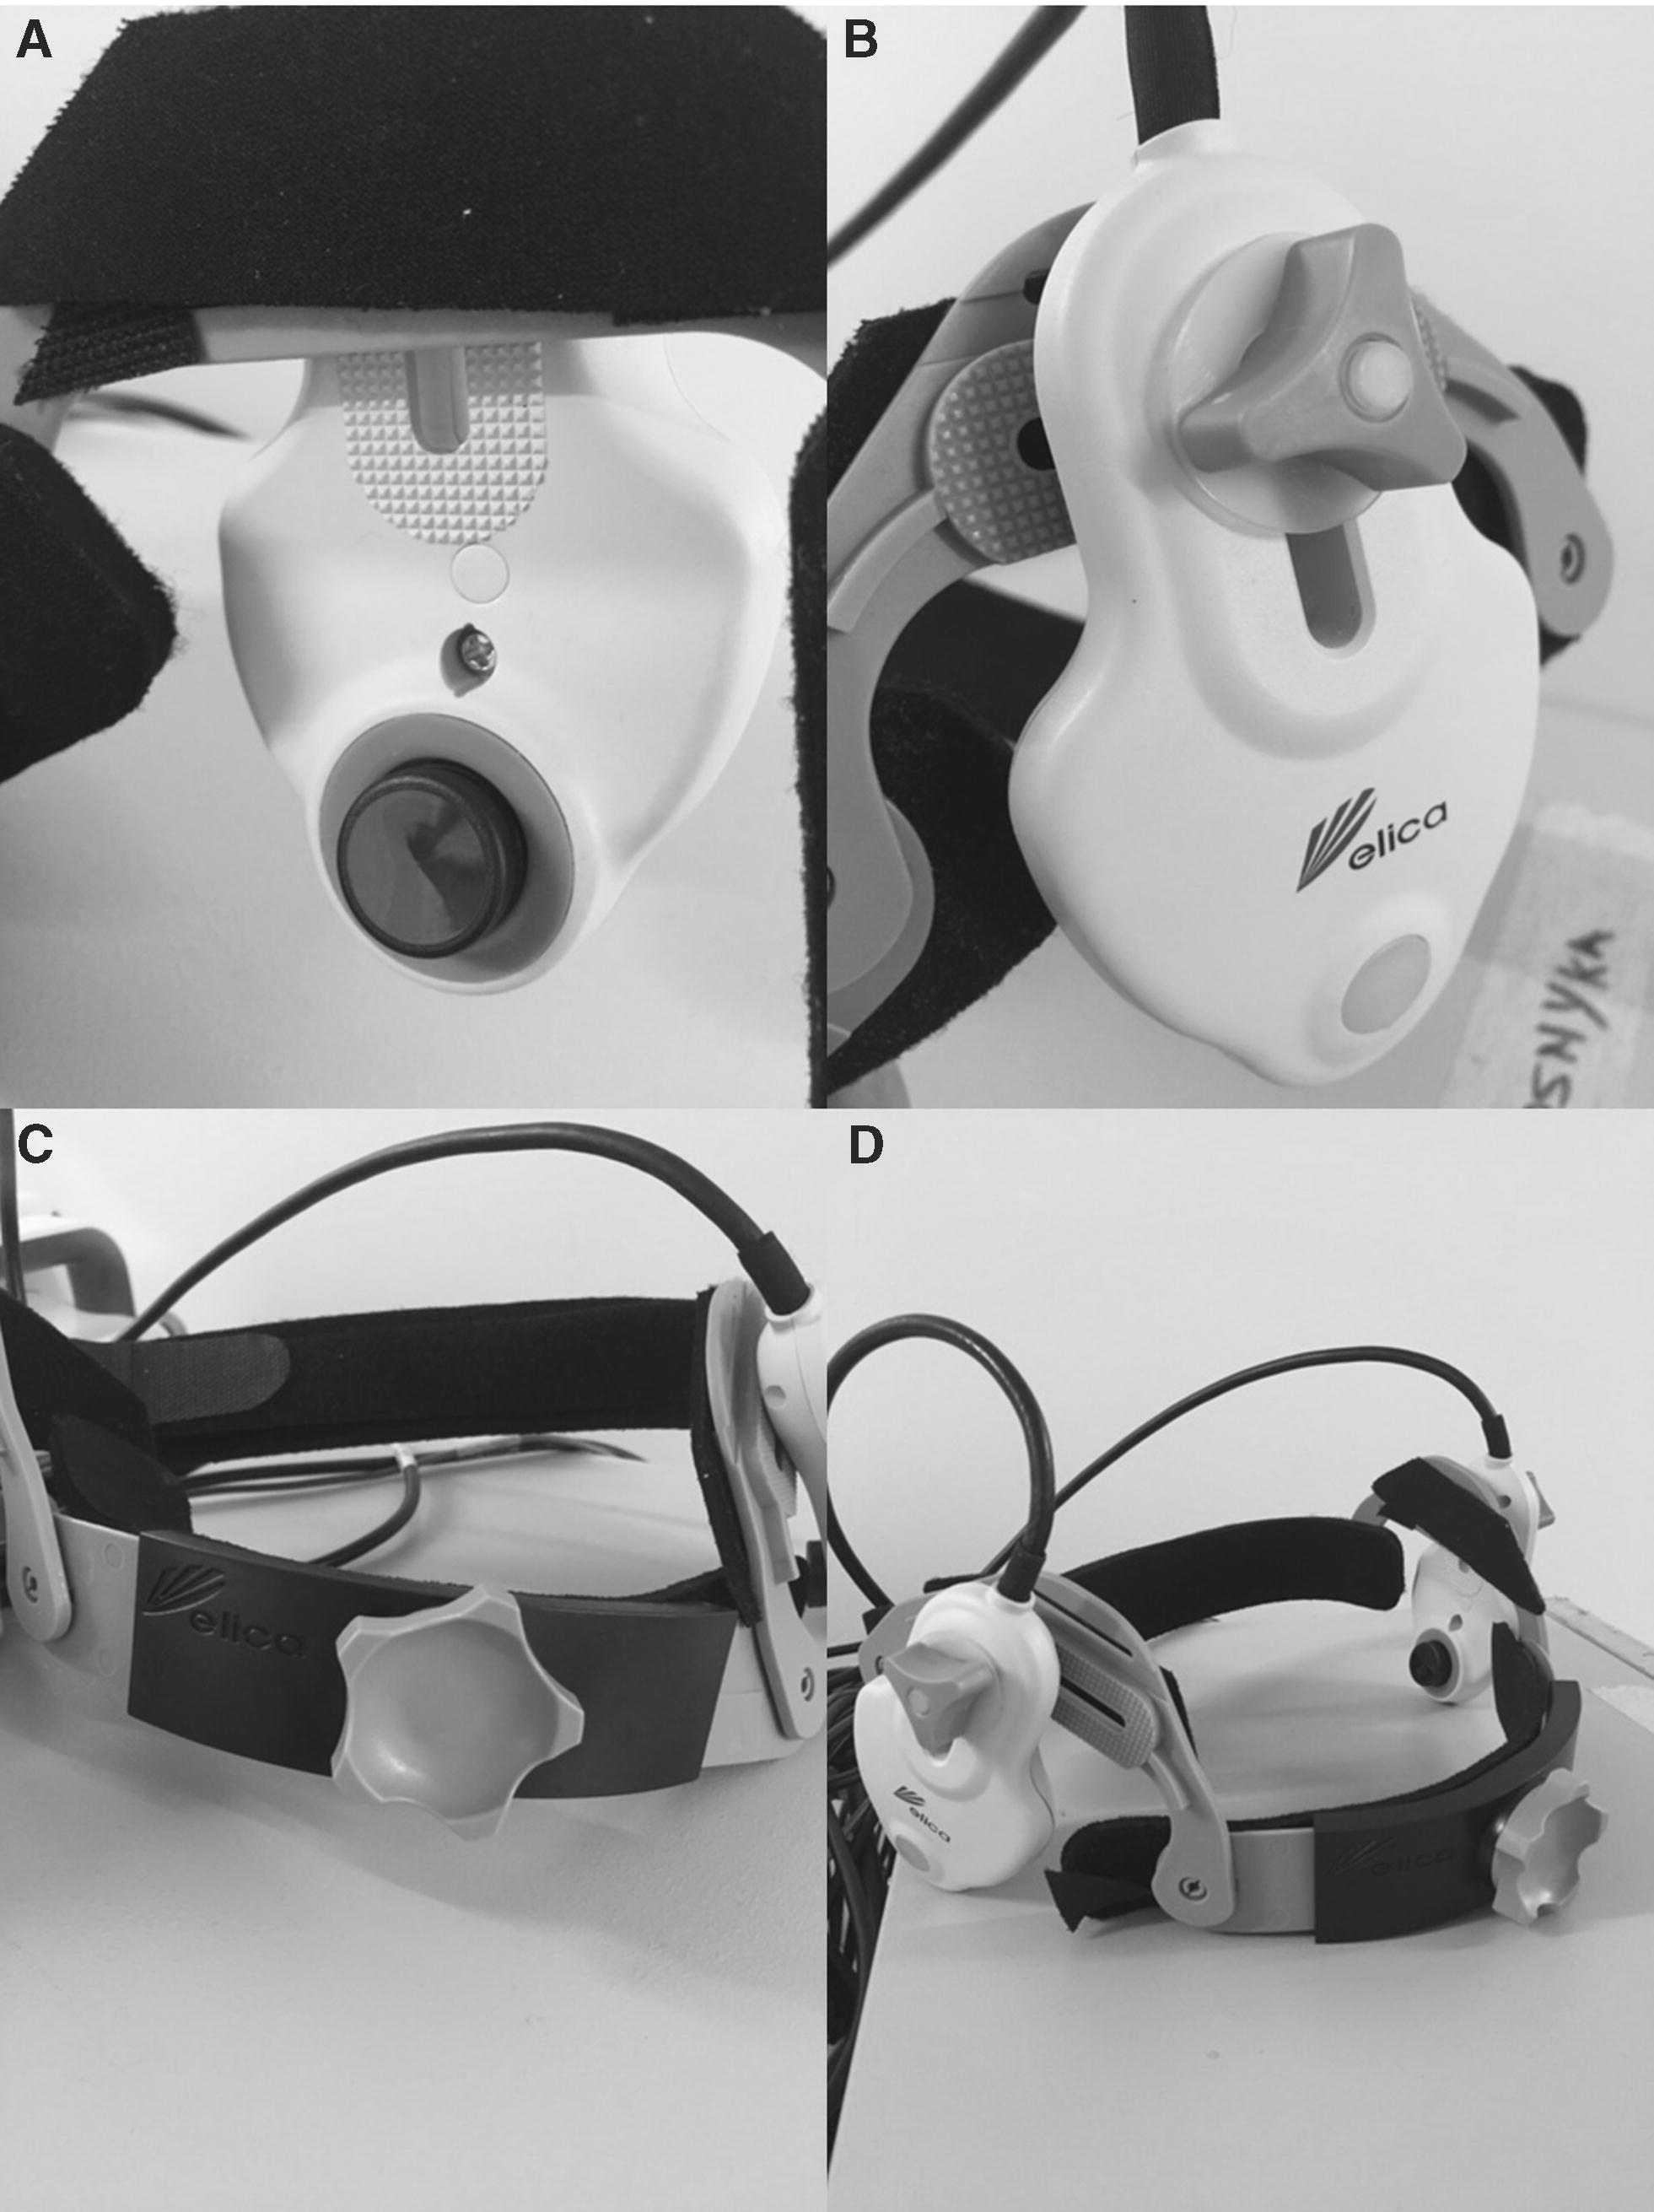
Delica EMS 9D robotic TCD probe and headband. *TCD* transcranial Doppler. A TCD probe (black circular object) held with robotic drive as one construct within plastic casing. Medial view—where probe contacts patient for transtemporal insonation of the middle cerebral artery. Neoprene pad is also seen on medial aspect of head-band. B Lateral view of robotic drive/TCD probe construct. Displays wing-nut attachment to head-band holder, via “inverted U-shaped” plastic bracket. C Anterior view of head-band displaying plastic component and wheel ratchet system for tightening. D Full view of head-band with bilateral robotic drive/TCD probes attached
